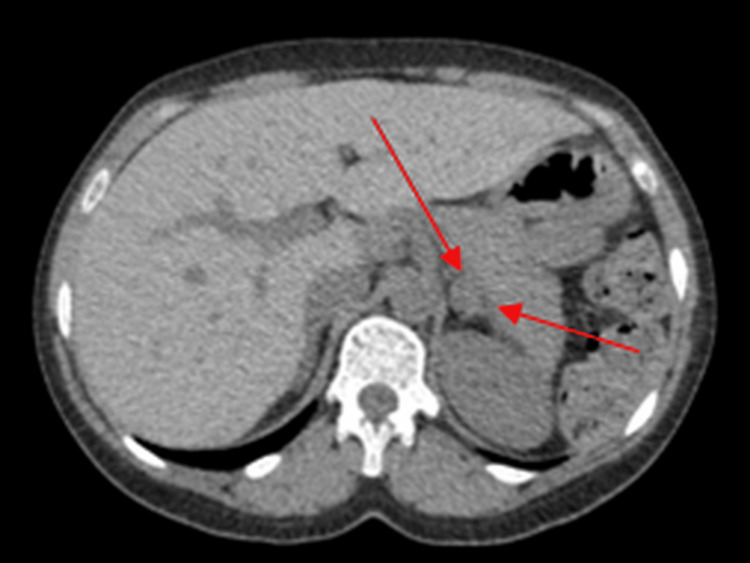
Initial computed tomography (CT) of the adrenal gland demonstrating a 1.2-cm adrenal mass (red arrows).
